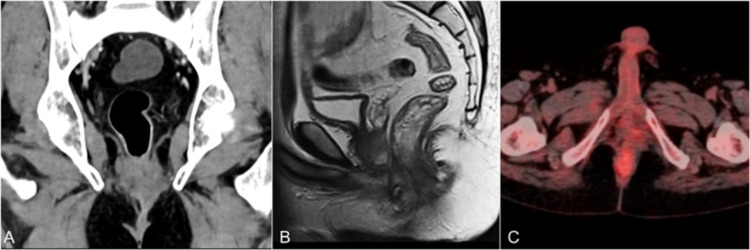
Posttreatment cross-sectional imaging (A) CT, (B) MRI, and (C) PET images showing resolution of the tumor. CT: computed tomography, MRI: magnetic resonance imaging, PET: positron emission tomography.
